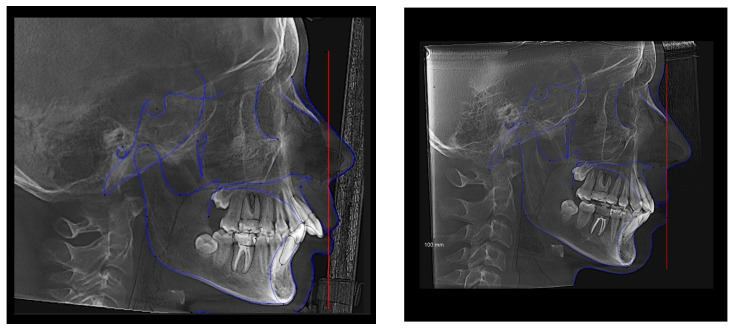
T0 and T1 cephalometric analysis from CBCT images using Dolphin software.
